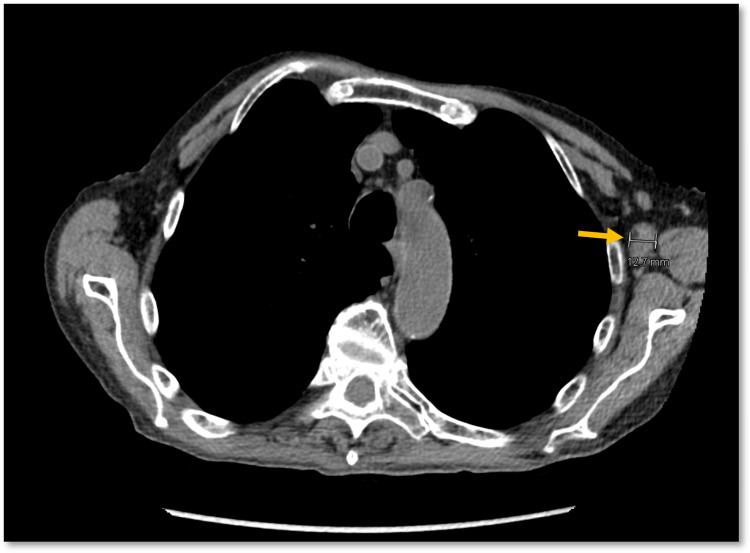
CT chest demonstrating left axillary lymphadenopathy The measurement bar (end of the yellow arrow) indicates that the largest diameter of the enlarged lymph node was 12.7 mm. CT, computed tomography
